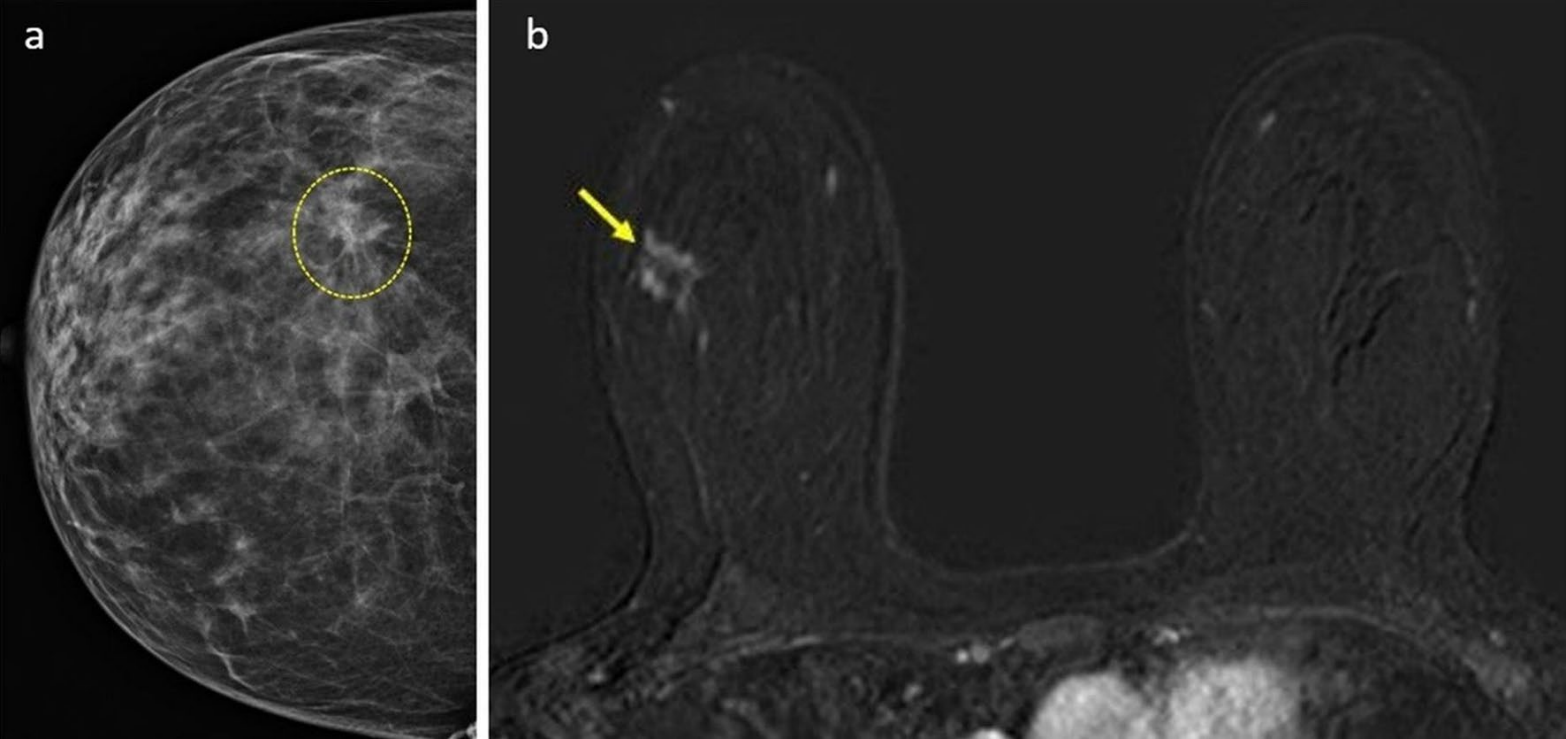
A 49-year-old woman underwent mammography for routine screening, revealing a suspicious area of architectural distortion (indicated by dashed yellow lines) in the outer quadrant of the right breast (a), in the area where distortion was observed on mammography, a lesion with irregular margins, rim-like, and inhomogeneous enhancement was identified on the dynamic contrast-enhanced subtraction MRI (b). The Kaiser score of the lesion was 7. Surgical excision pathology confirmed the diagnosis of invasive ductal carcinoma.
